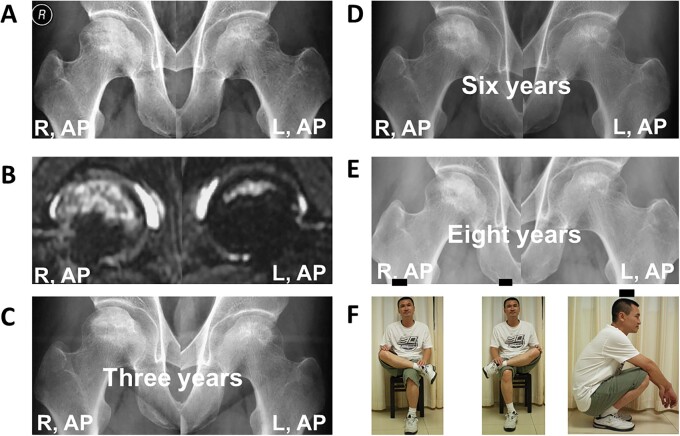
A 50-year-old man (Patient 6) who has idiopathic necrosis of the femoral head. (A) Frog-leg lateral radiographs and anteroposterior radiographs show bilateral hips with stage II disease at his initial diagnosis, the right and left are type A. (B) MRI image of the suffered hip obtained at initial diagnosis. (C, D and E) The three years, the sixth year and the eighth year, the patient was free from symptoms and had no radiographic progression of the double femoral head. (F) Until eight years later, the patient was free from symptoms and had good function.
